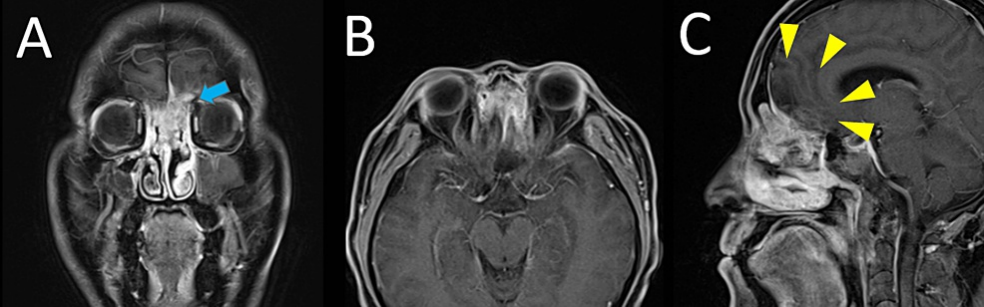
Contrast-enhanced MRI after three cycles of IC Coronal (A), axial (B), and sagittal (C) planes: the tumor decreased in size from 67 mm × 32 mm × 55 mm to 38 mm × 28 mm × 33 mm. The abnormal enhancing areas in the frontal lobe white matter also tended to disappear (C: yellow arrowhead). The tumor had extensive contact with the left frontal base (A: blue arrow).
